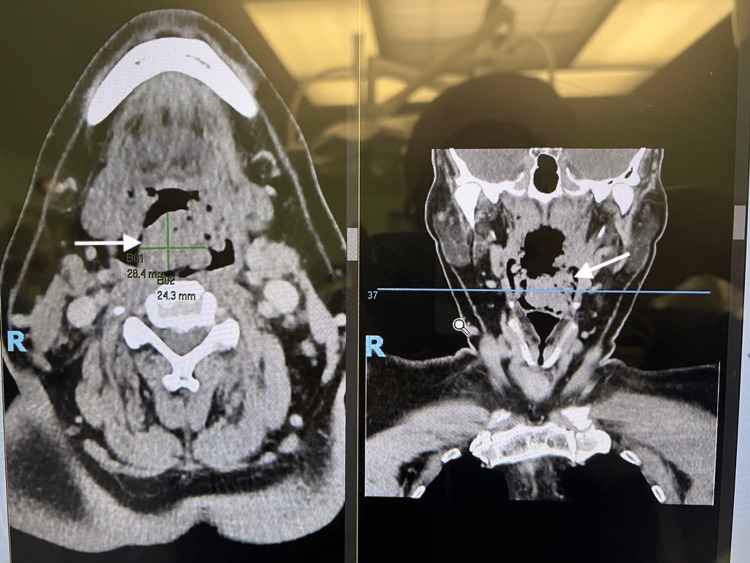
Case 3: CT neck showing adenoidal hyperplasia with enlargement of the central nasopharyngeal soft tissues and bilateral palatine tonsils (denoted by white arrows).
